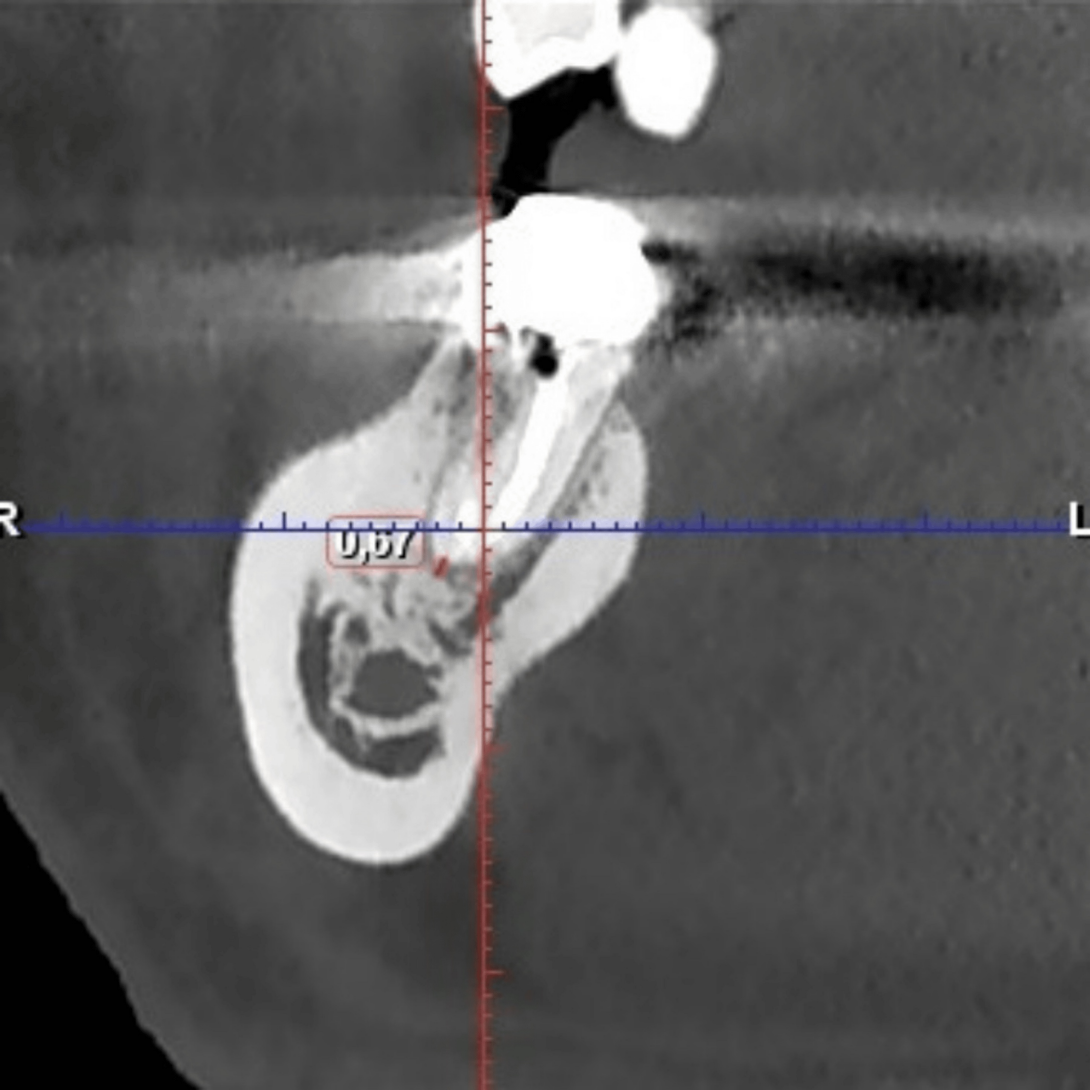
CBCT image of the mesial root of tooth 47 showing measurements of the apical defect, in coronal section, after three months. CBCT, cone beam computed tomography.
